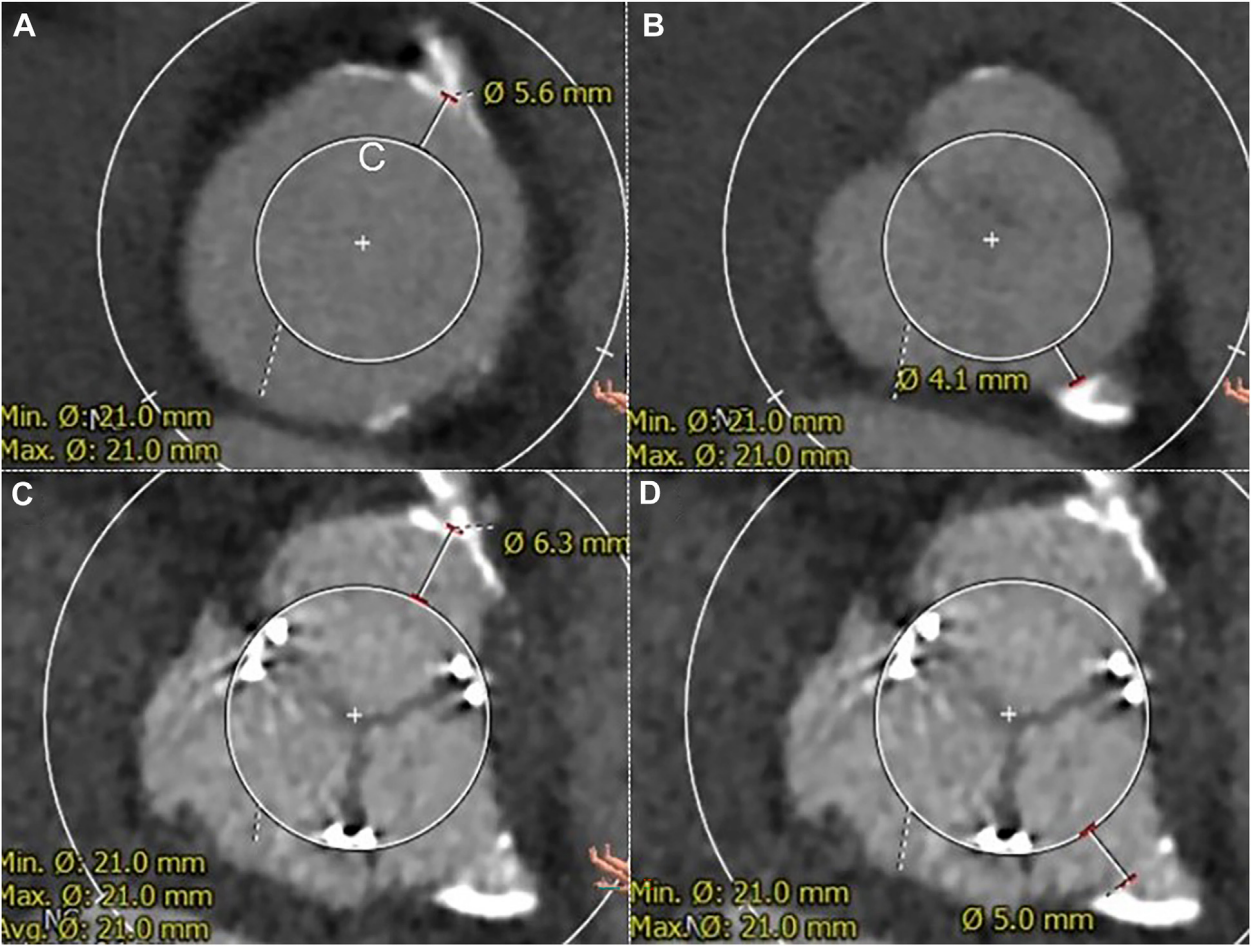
Preoperative computed tomography showing that the virtual distances from the surgical valve to the (A) right and (B) left coronary arteries are 5.6 mm and 4.1 mm, respectively. Postoperative computed tomography showing that the virtual distances from the surgical valve to the (D) right and (E) left coronary arteries are 6.3 mm and 5.0 mm, respectively. (Avg., average; Max., maximum; Min., minimum.)
